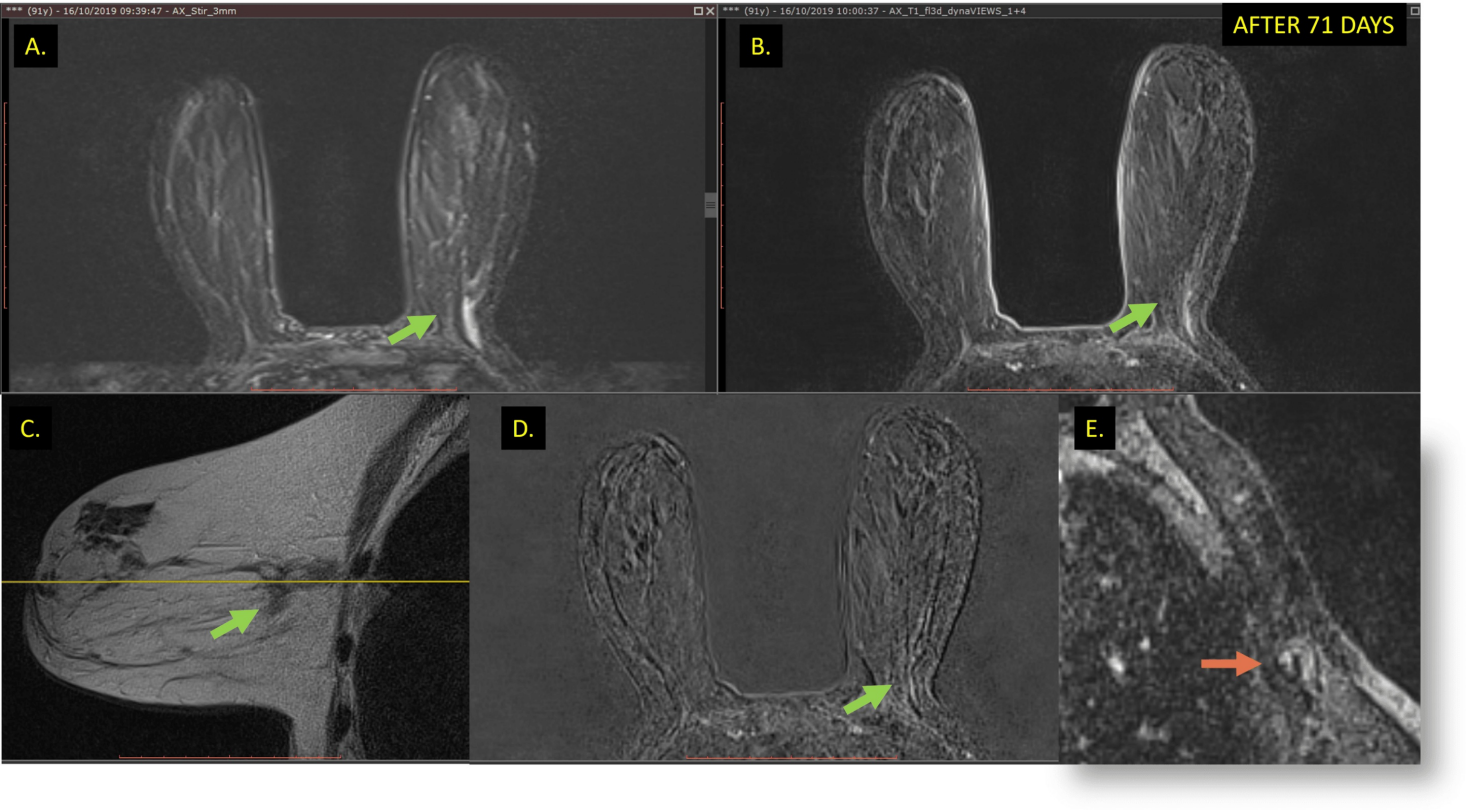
Breast MRI after biopsy. Breast MRI showing tumor remission compatible with a complete radiological response (green arrow) (A, B, C and D) (orange arrow) and lymph node (E) (orange arrow) regression.
